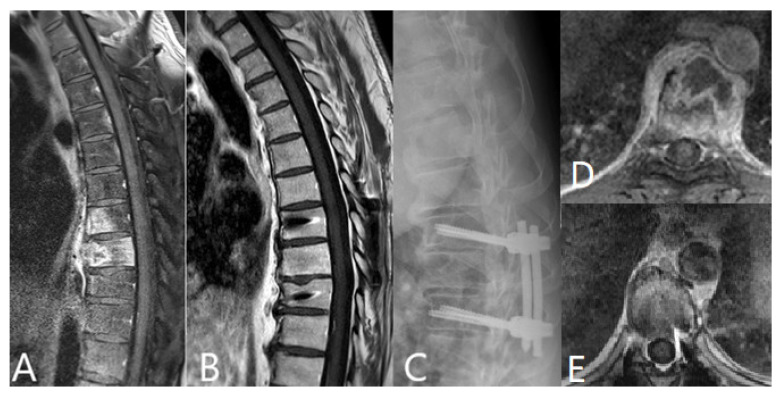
44-year-old male patient with spondylodiscitis of the thoracic spine due to an infection with *Mycobacterium tuberculosis*. (A). preoperative T1 post-contrast MRI of the thoracic spine shows spondylodiscitis in Th 7/8 and Th 8/9. (B). postoperative T1 post-contrast of the thoracic spine shows the complete resolution of the infection 6 months following surgery. (C). postoperative X-ray of the thoracic spine shows the spinal construct with screws and rods in T7-T9. (D). Axial T1-weighted post-contrast MRI of the spine at Th7 level shows infection predominantly in ventral portion of the vertebral body. (E). T1-weighted post-contrast MRI of the spine at Th7 level 6 months following surgery shows complete resolution of the infection.
